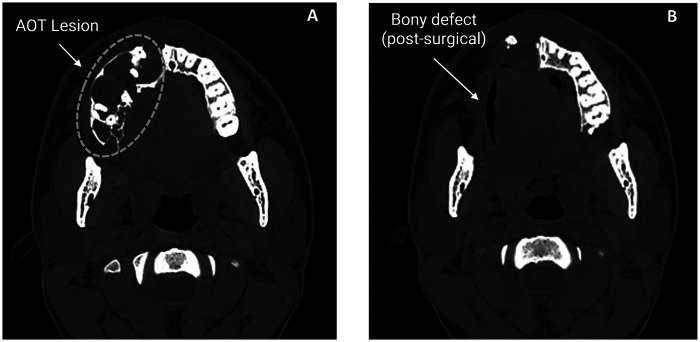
Axial computed tomography images. (A) Preoperative scan showing a multilocular lesion in the right hemimaxilla with a “soap bubble” appearance and internal calcifications (outlined in green), involving teeth 1.5, 1.6, 1.7, and 1.8, and extending across the midline to involve the left maxilla. (B) Postoperative scan at 5-month follow-up demonstrating absence of the lesion and postoperative changes in the right maxillary region.
